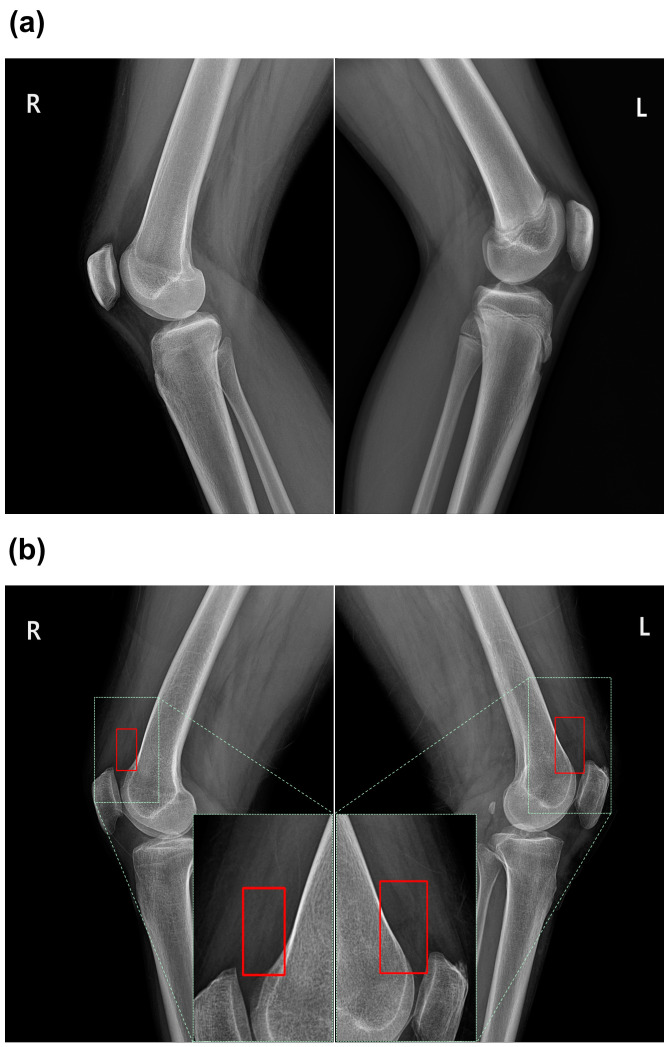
Sample X-ray images of patients with knee: (a) normal case; (b) effusion case (the red bounding box indicates the area of effusion).
